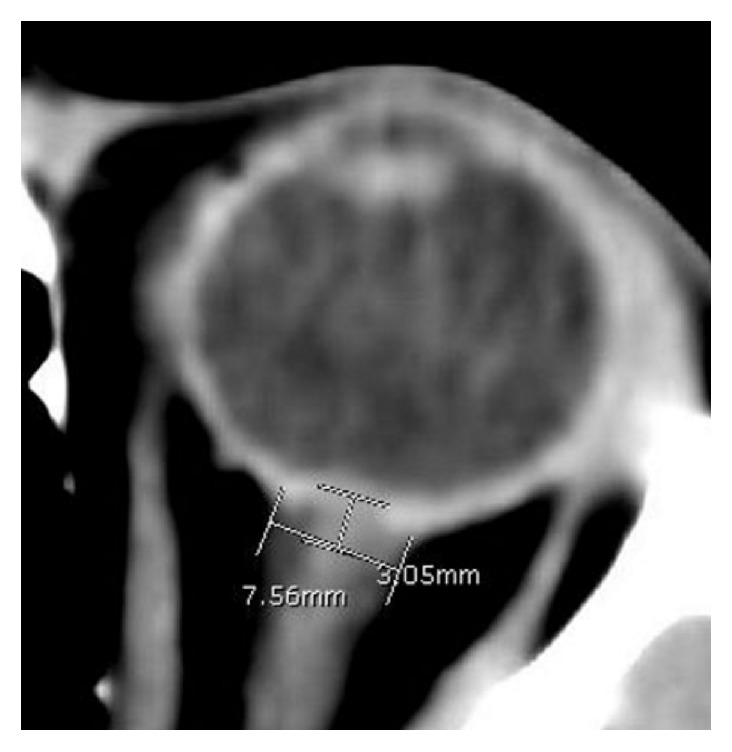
Showing a CT scan with an ONSD of 7.56 mm measured 3 mm posterior to the globe.
